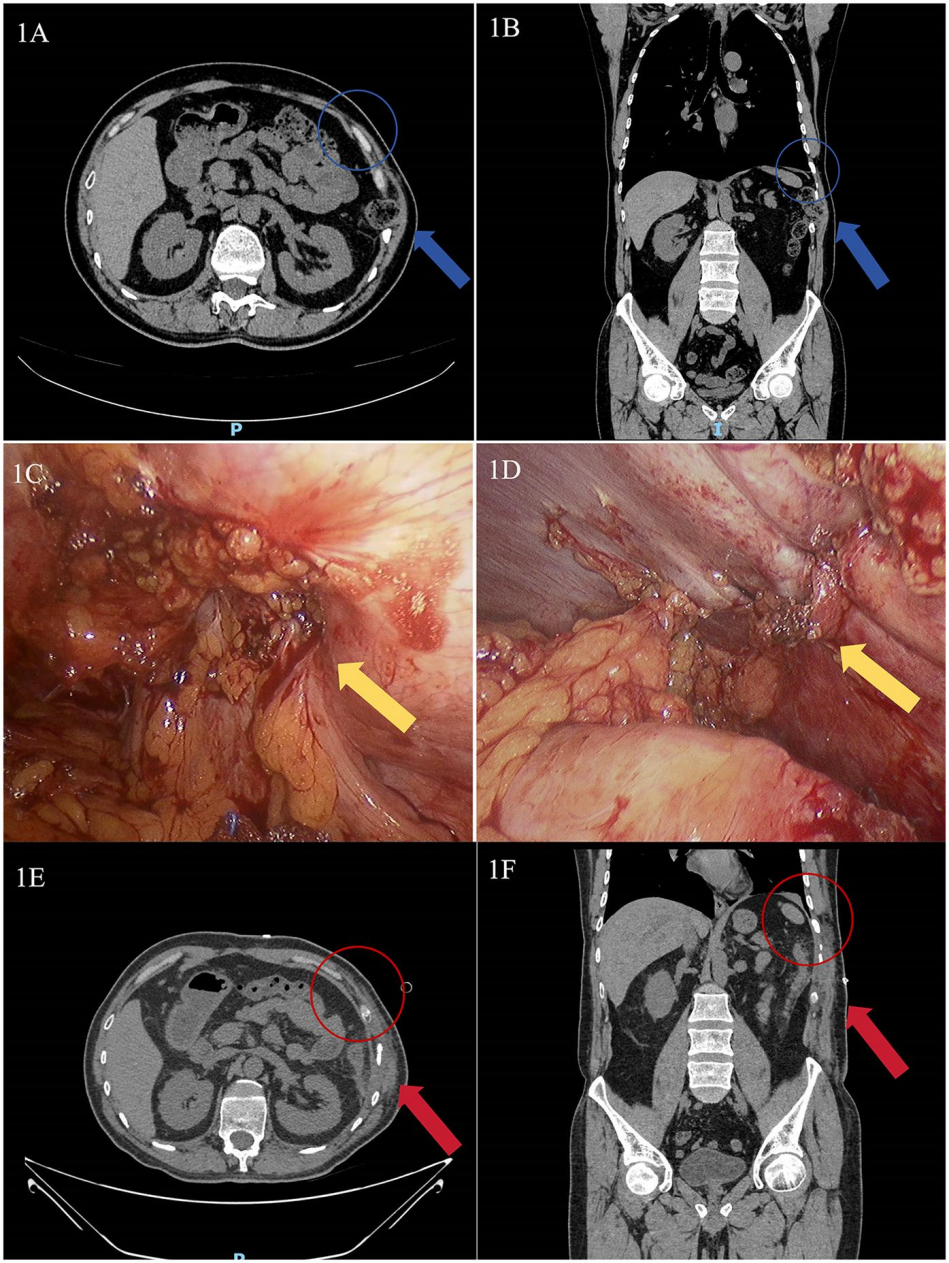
Abdominal CT and intraoperative images of the patient treated in our hospital. (A) Preoperative axial abdominal CT image (The blue arrow indicates the location of the intercostal hernia, the blue circle demarcates the area of diaphragmatic rupture); (B) Preoperative coronal abdominal CT image (The blue arrow indicates the location of the intercostal hernia, the blue circle demarcates the area of diaphragmatic rupture); (C) Intraoperative view of the hernia sac (The yellow arrow indicates the site of defect, with the colon herniated through it); (D) Intraoperative view after diaphragmatic defect repair (The yellow arrow denotes the post-repair site. The defect was closed with interrupted sutures, and no mesh was deployed); (E) Postoperative axial abdominal CT image (The red arrow indicates the successfully repaired defect with resolution of the hernial sac; the red circle demonstrates the restored continuity of the diaphragm); (F) Postoperative coronal abdominal CT image (The red arrow indicates the successfully repaired defect with resolution of the hernial sac; the red circle demonstrates the restored continuity of the diaphragm).
